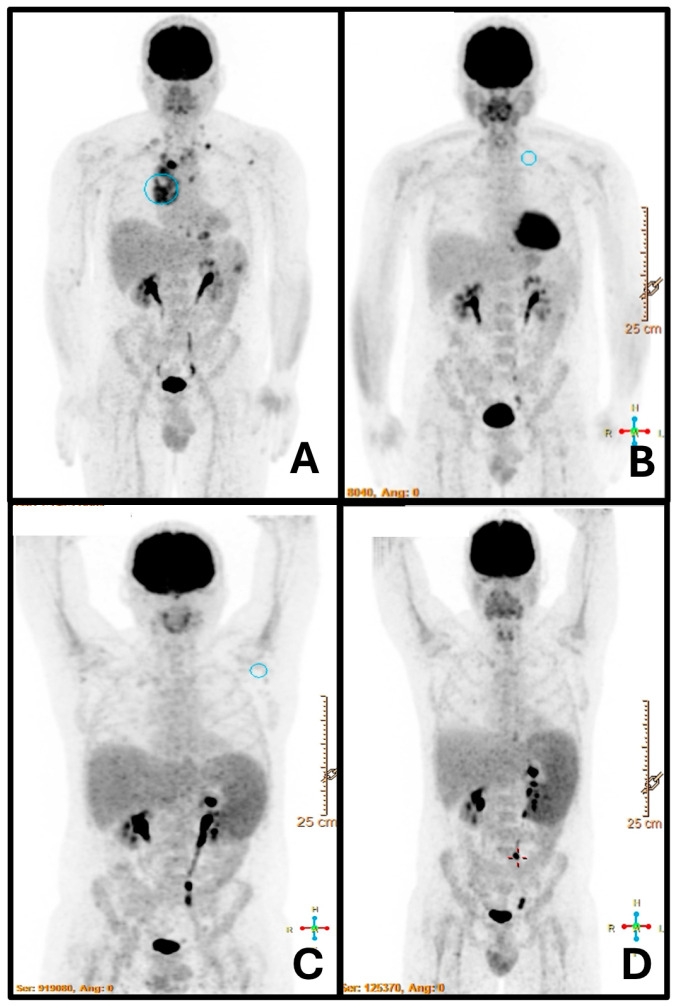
PET-TC at cHL diagnosis (A): increased glycolytic metabolism observed in multiple lymph nodes, precisely above the diaphragm, at the scapular level, and a notable lesion in the spleen without splenomegaly. After completing two cycles of immuno-chemotherapy (B), a complete response was achieved, and no evident lesions were observed at the spleen level. PET-CT performed one month post-treatment (C) revealed a homogeneous splenomegaly of 18 cm with no focal lesions (an abdominal ultrasound confirmed the absence of spleen lesions). Two months later, imaging confirmed an increase in splenomegaly measuring 20 cm, without any other findings (D), when a diagnosis of VL was established.
